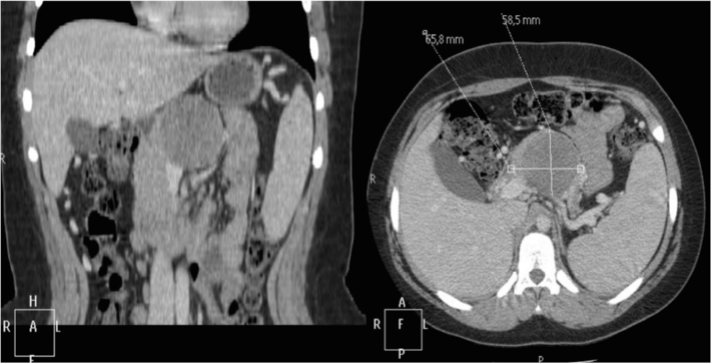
Computed tomography scans showing a cystic and solid mass 5.2 × 5.5 × 5.2 cm^3^ arising from the trunk of the pancreas
